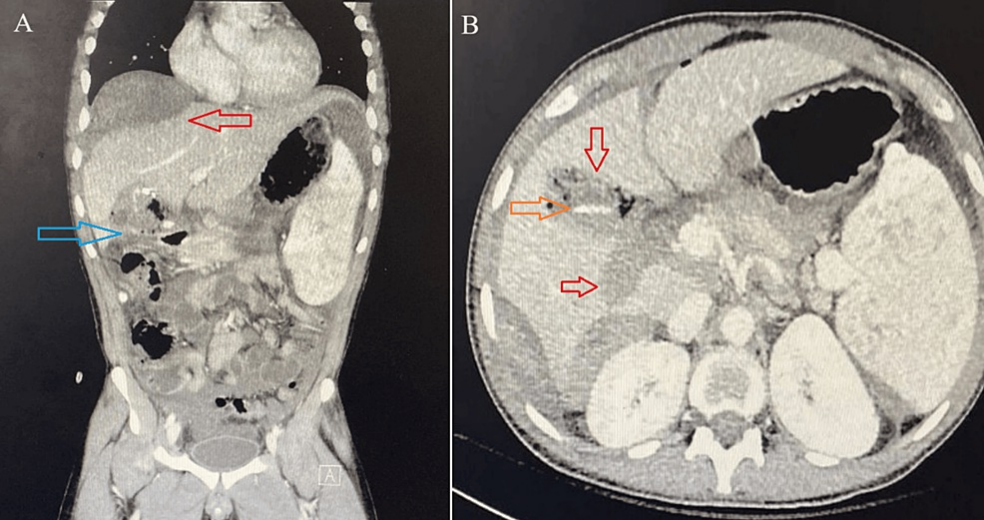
Contrast-enhanced CT scan of the abdomen. A) Coronal view showing large hepatic subcapsular hematoma appearing hyperdense with areas of contrast extravasation on arterial phase, which progress in venous phase (red arrow) and surgical bed collection (blue arrow). B) Axial view showing surgical bed collection with multiple air foci which related to previous surgery (red arrow), drain in the surgical bed (orange arrow). Incidental finding of splenomegaly measuring about 20 cm.
